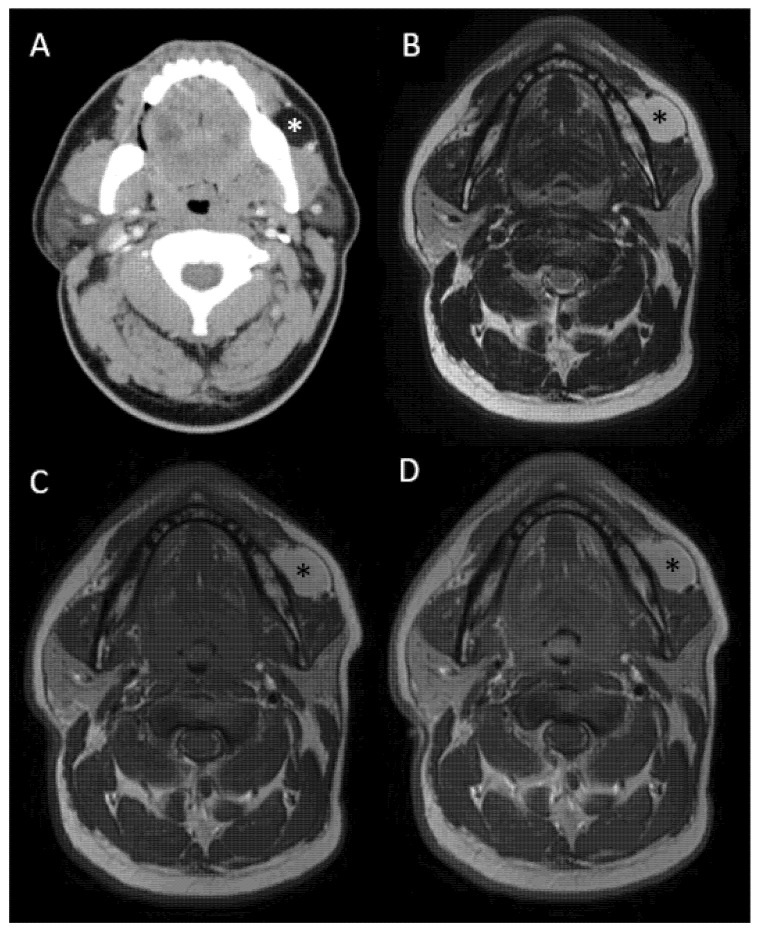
(A) Post contrast CT scan showing a homogeneous, low-attenuation mass (2.2 × 4.1 × 2.0 cm) in the left buccal space (asterisk). (B) On T1- and (C) T2-weighted MRI sequences, the mass had a high signal (asterisk) and (D) did not enhance after gadolinium administration on the T1-weighted, fat suppressed, post-contrast sequence (asterisk).
